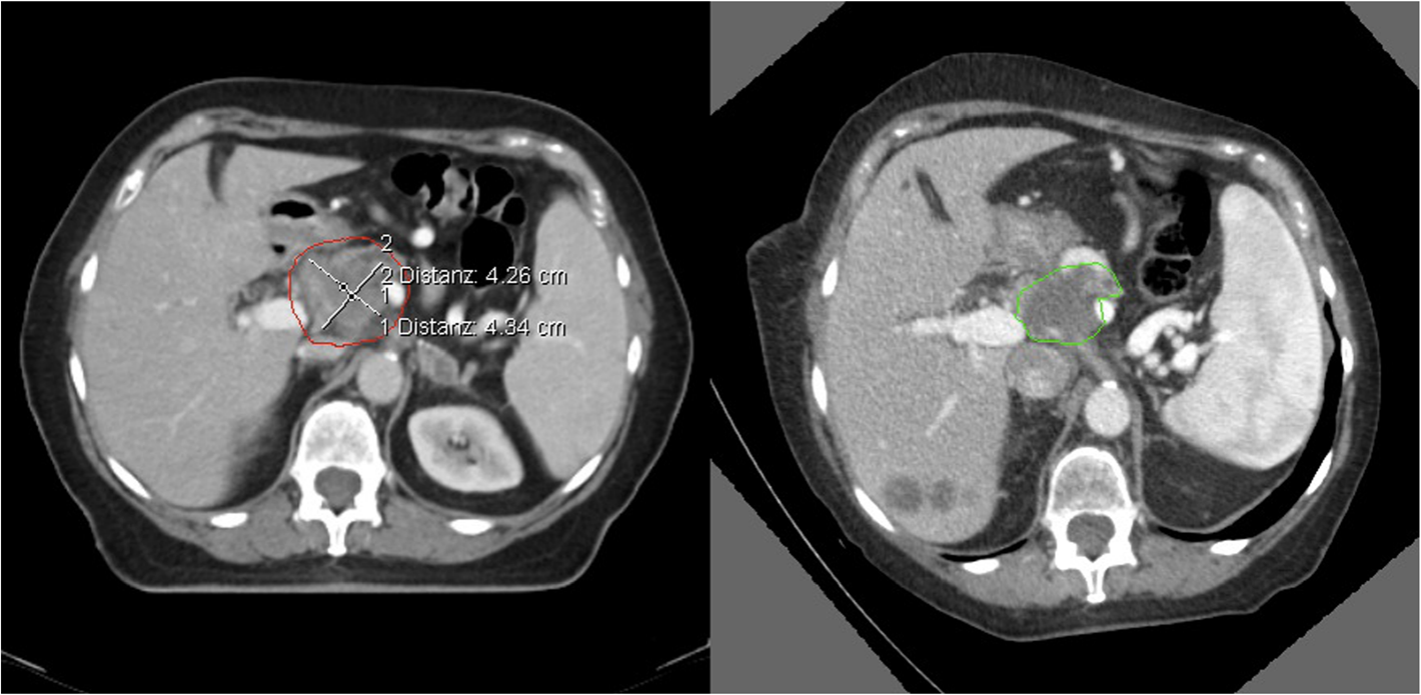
CT scans of patient 3; left: planning CT, right: follow-up CT after automatic registration with rotation and severe deformation.
